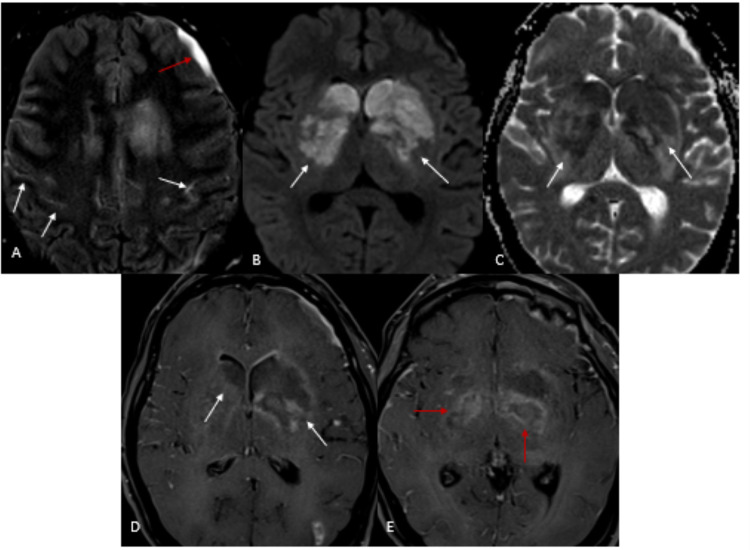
Intracranial extension of the mucormycosis (A) T2 FLAIR post-gadolinium: leptomeningeal enhancement and FLAIR-nonsuppression (white arrows) and subdural collections (red arrow); (B)-(C) DWI and ADC: diffusion restriction in the deep nuclei bilaterally (white arrows); (D)-(E) T1 post-gadolinium MPRAGE: large necrotic foci in the left greater than right basal ganglia (white arrows) and the hypothalamus (red arrows). FLAIR: fluid-attenuated inversion recovery; MPRAGE: magnetization-prepared rapid acquisition gradient echo; DWI: diffusion weighted imaging; ADC: apparent diffusion coefficient
